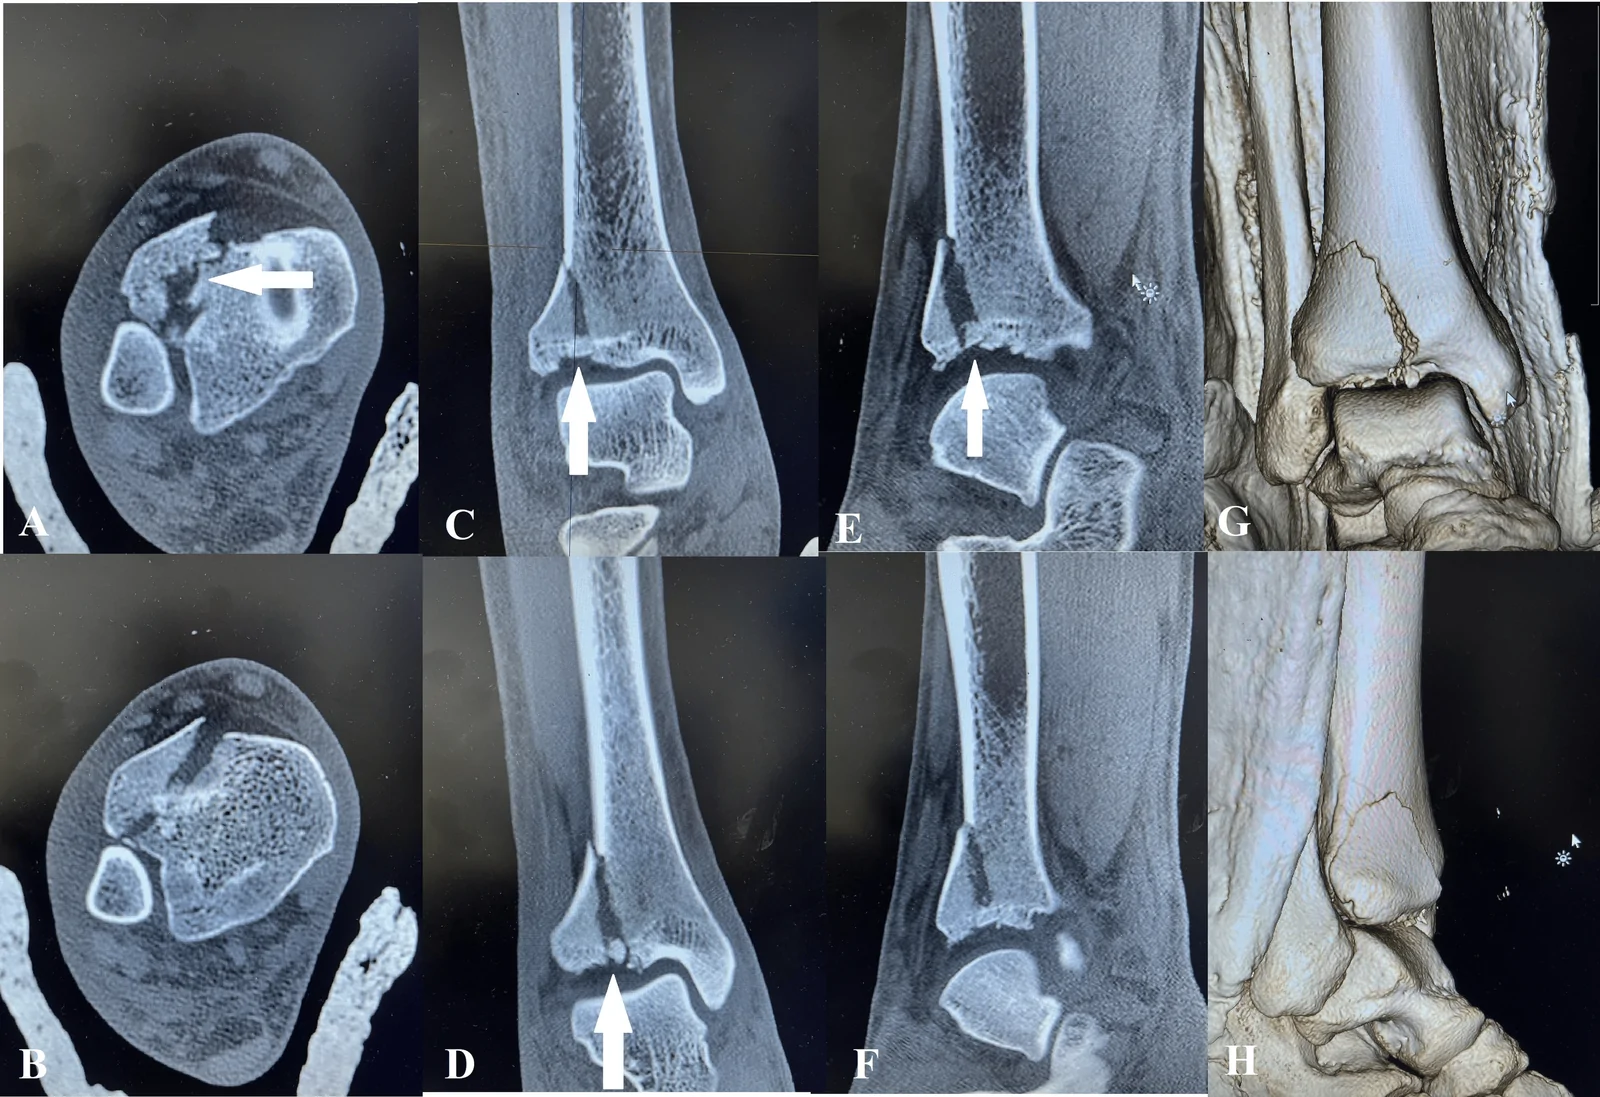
Initial CT scan. (A,B) Axial, (C, D) coronal, (E, F) sagittal, and (G, H) three-dimensional reconstruction CT scan cuts showing an isolated Chaput fracture with displacement greater than 2 mm and an associated articular step-off with incarcerated articular fracture piece (arrow).
